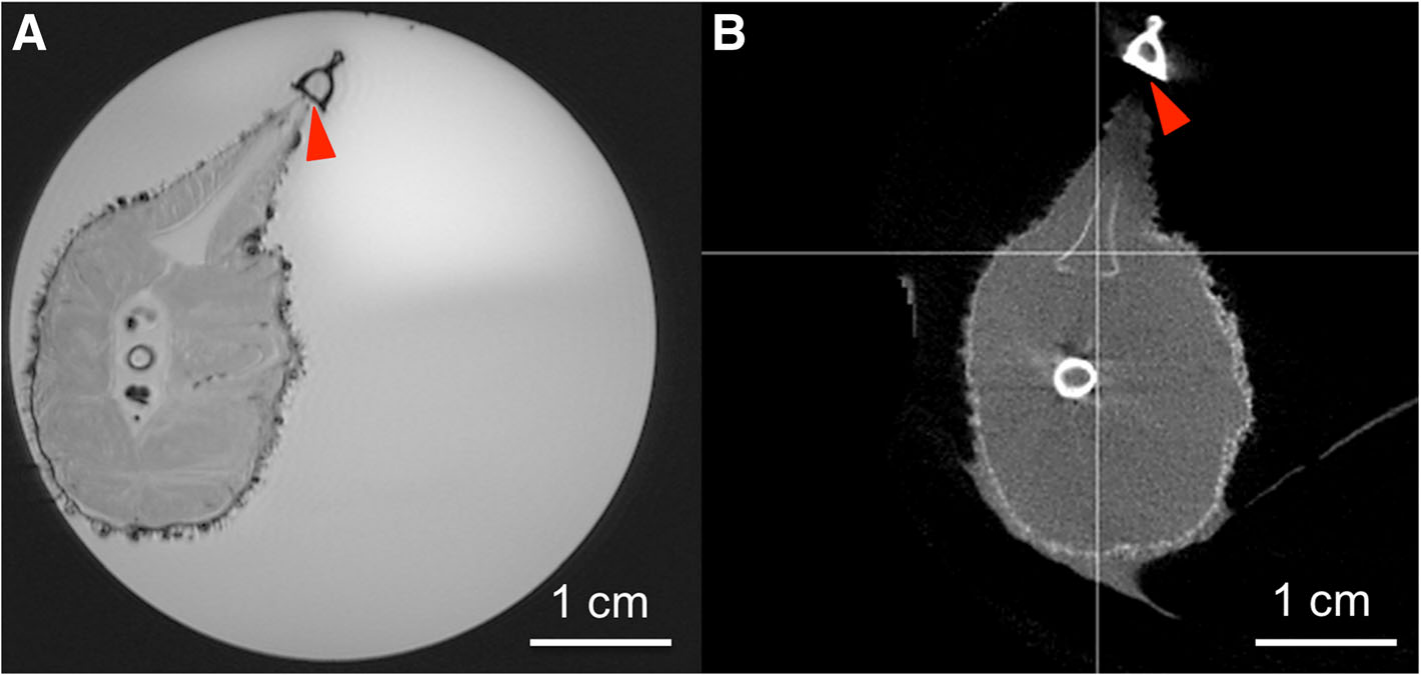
Spine structure. Spine structure analyses by MRI image showing any evidence of soft tissue at the posterior side of the spine (red arrowhead) (a). Internal spine structure analyses by micro CT scan showing any evidence of a duct/canal at the posterior side of the spine hard tissue (calcified structure), red arrowhead showing the calcified tissue at the posterior side of the spine without any duct/canal (b). In both analyses, the only hollow on the spine, at the center, is filled with cartilaginous matrix
